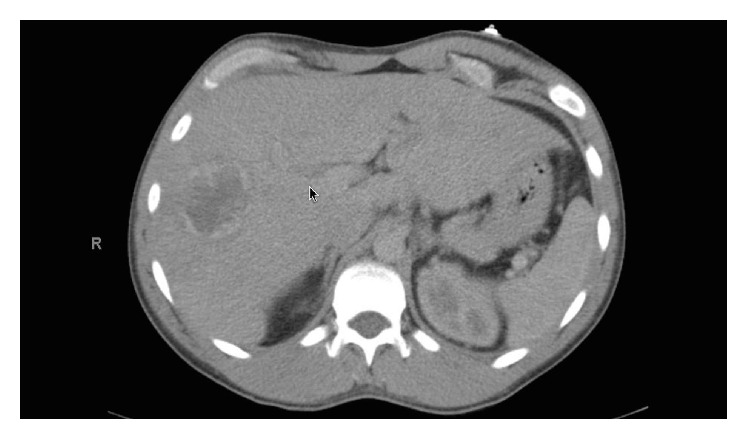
CT of the abdomen, revealing a single hypodense lesion with irregular foci and wall thickening in the right hepatic lobe.
